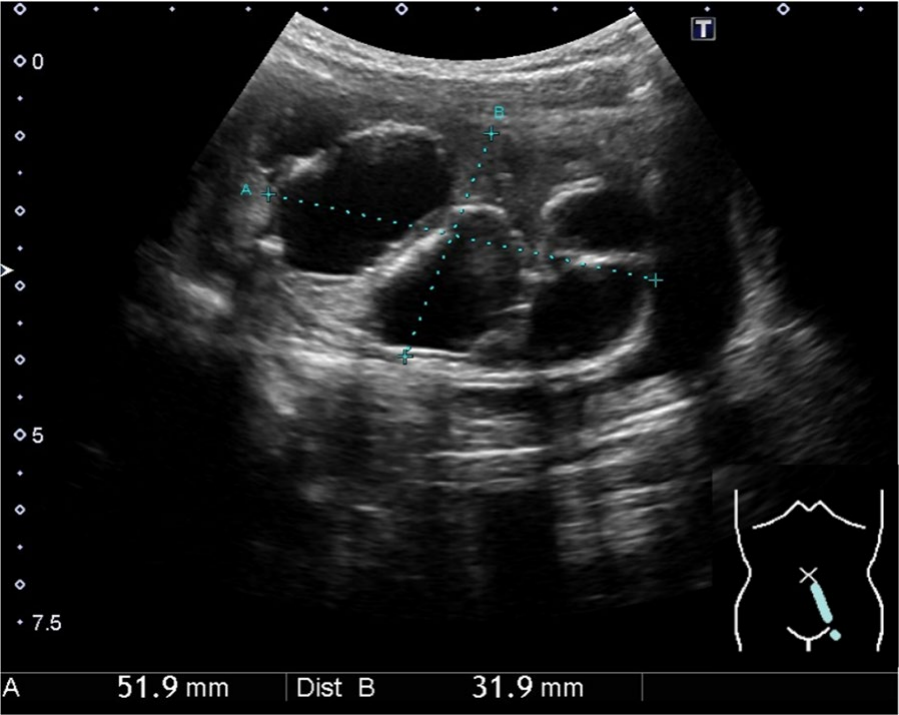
Abdominal ultrasonography findings. Abdominal ultrasonography revealed a multilocular cystic lesion to the left of the bladder, measuring 50 × 30 mm
